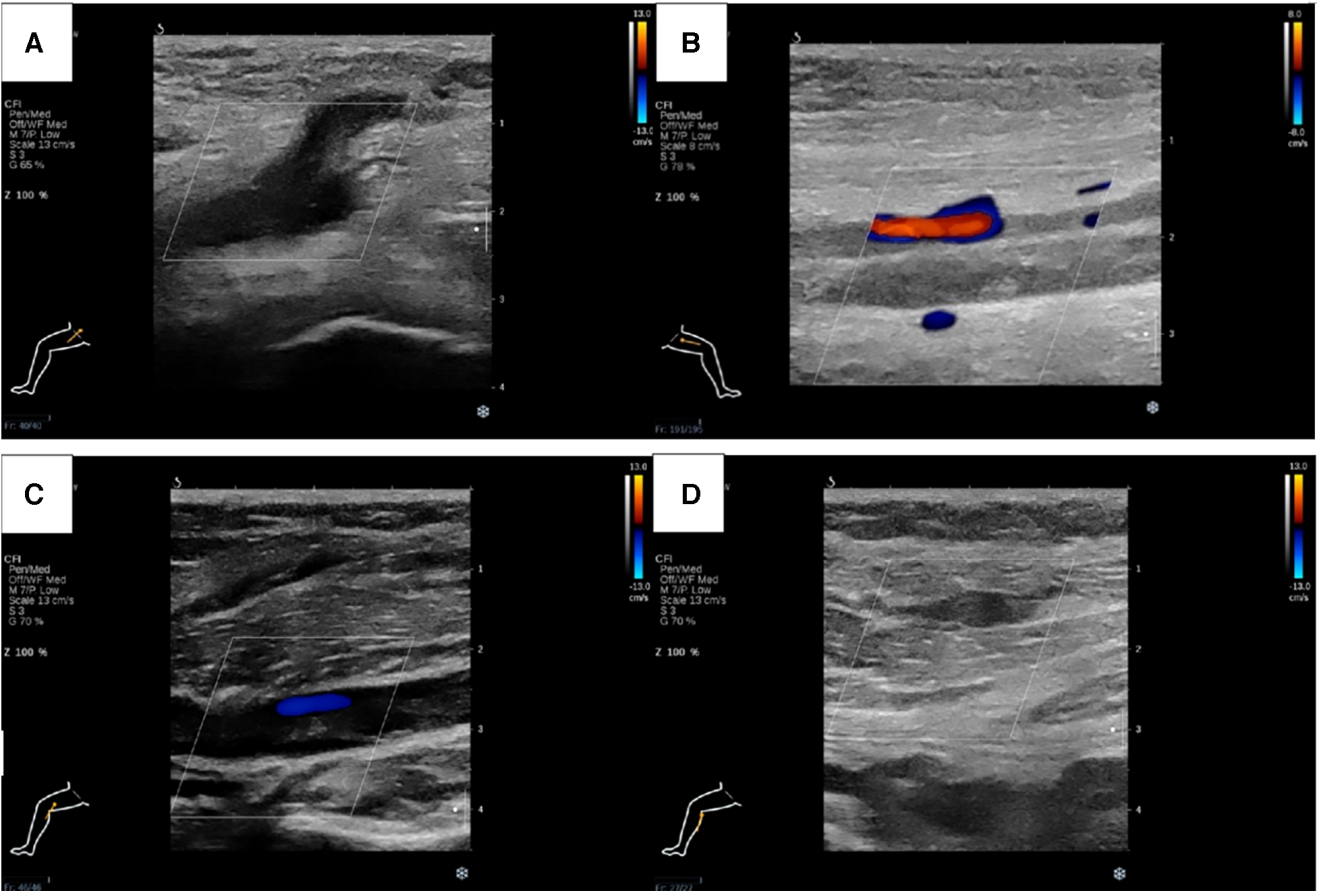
Lower extremity ultrasound demonstrated occlusive embolism of right common femoral vein (A), bilateral superficial femoral vein (B), right popliteal vein (C) and calf muscular vein (D).
